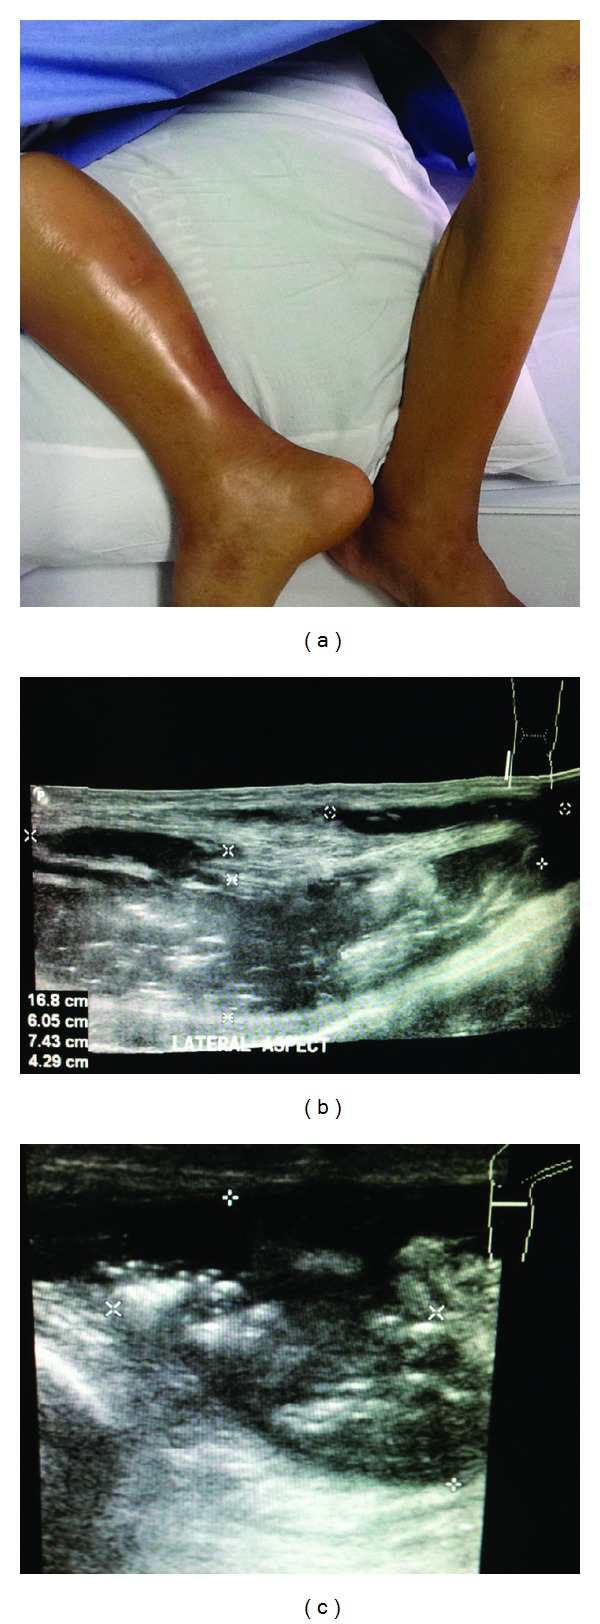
(a) After antimicrobial treatment, there was progressive swelling of right knee extended downward to the calf area, (b, c) ultrasonography of right leg discovered large space of air-filled, heterogeneous multiloculated peri- and intramuscular abscesses, sized 5.4 × 8.6 × 17 centimeters in diameter, extended from popliteal area to ankle level.
